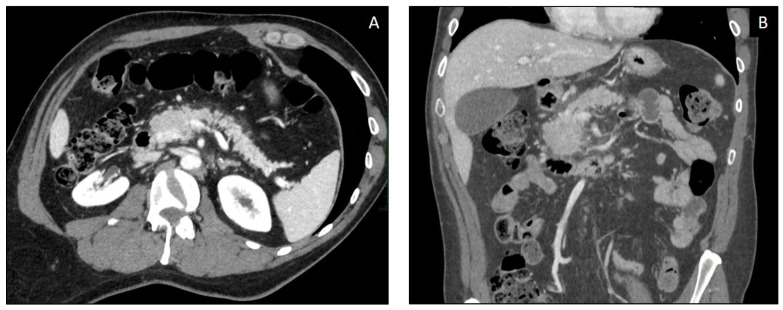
50-year-old patient with melanoma treated with Ipilimumab. (A,B) Axial and coronal post-contrast CT images, demonstrating acute pancreatitis after 10 weeks of ICI administration, with parenchymal enlargement and inhomogeneous enhancement with diffuse hypodensity at the level of the head and uncinate process, associated with adjacent fat stranding.
